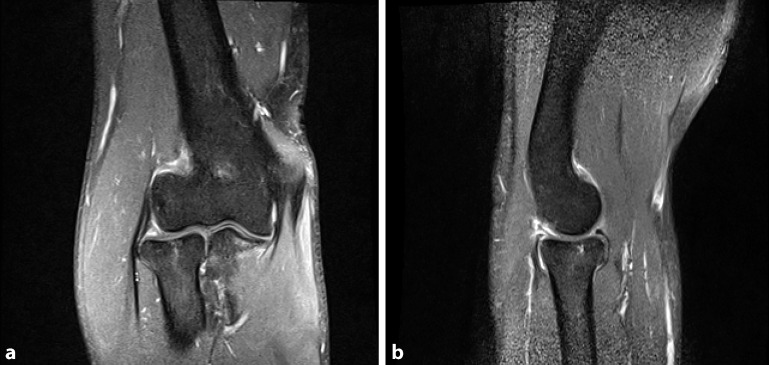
Magnetic resonance imaging of Mason I radial head fracture and absence of soft tissue damage
